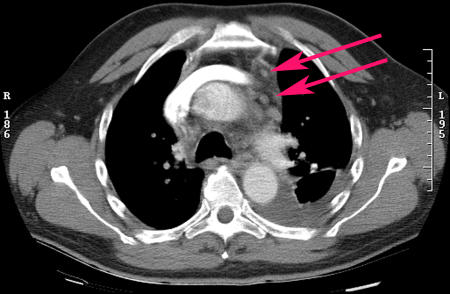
CT of the Chest during the Third Hospitalization The CT shows mediastinal lymphadenopathy (pink arrows) and left pleural effusion.
